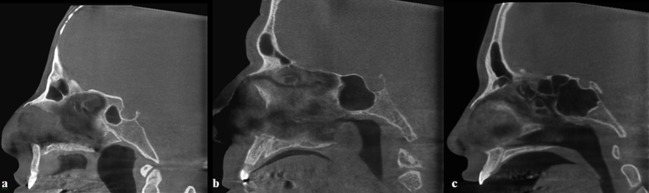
Sagittal CBCT section showing type II, III, and IV pneumatization of the sphenoid sinus  a: Type II,  b: Type III, c: Type IV
